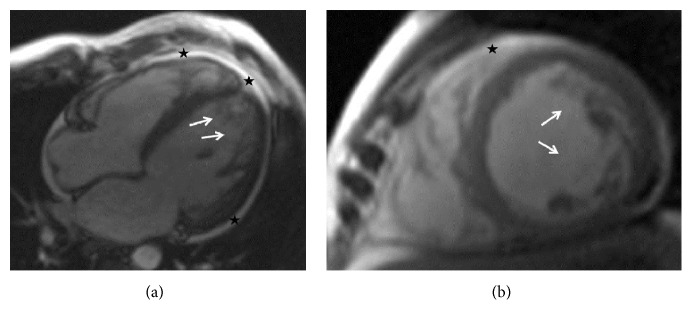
Cardiac MRI, cine mode, showing apical hypertrabeculation (arrows) and circumference pericardial effusion (asterisk) ((a) 4-chamber view, (b) midventricular 2-chamber view).
